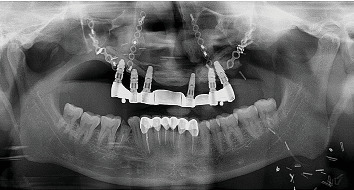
Orthopantomogram during the follow-up visit.
